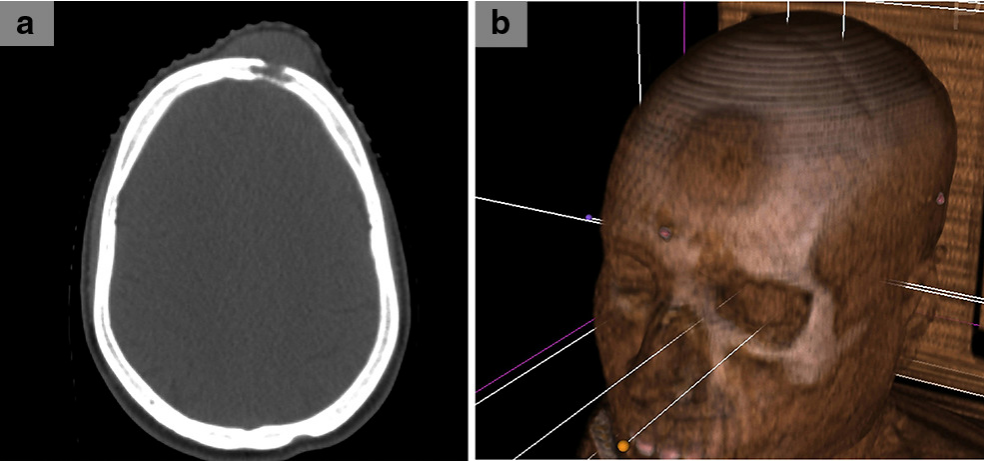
Axial CT slice (a) demonstrating the frontal bone metastasis involving both the outer and inner tables of the bone; three-dimensional reconstruction (b) of the lesion demonstrating the location within the frontal bone.
